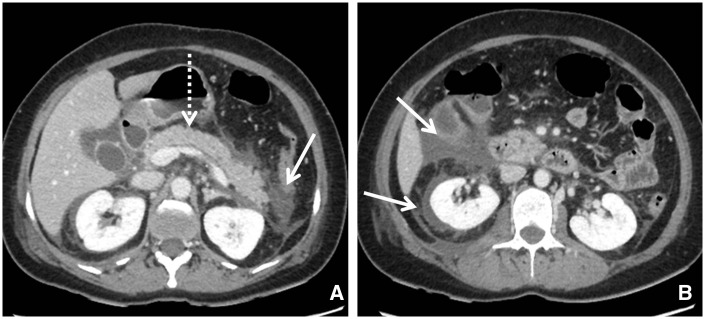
(A and B) Axial contrast-enhanced CT scan in a 53-year-old male with acute interstitial pancreatitis and acute peripancreatic fluid collection (APFC) in the peripancreatic, right anterior pararenal and perirenal spaces (arrows). The pancreas shows homogeneous enhancement (dashed arrow). APFC has fluid density without an encapsulating wall.
